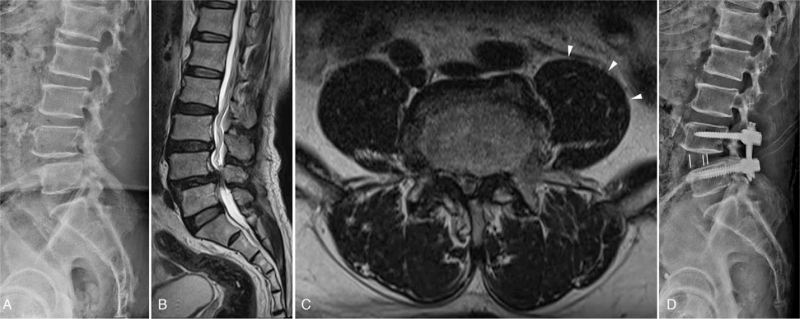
Perioperative imaging for the first operation. (A) Lateral radiographs of the lumbar spine demonstrate L4-5 grade I spondylolisthesis. (B) Lumbar MRI indicates L4-5 stenosis. (C) Preoperative lumbar MRI indicates enlarged left iliopsoas muscle (white arrows). (D) Postoperative radiography shows a relatively anterior location of the cage. MRI = magnetic resonance imaging.
